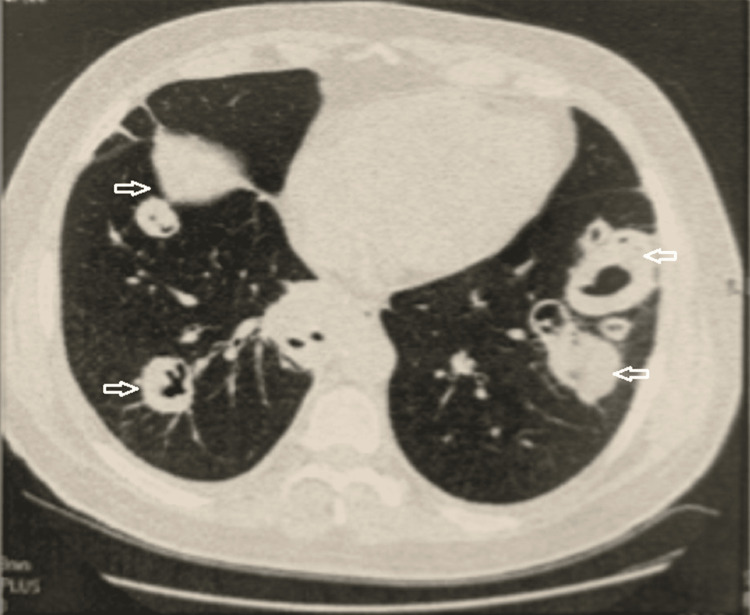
Axial chest CT scan demonstrating multiple bilateral cavitary pulmonary nodules with irregular margins, suggestive of necrosis. Arrows indicate representative cavitary nodules with thick, irregular walls of variable size.
